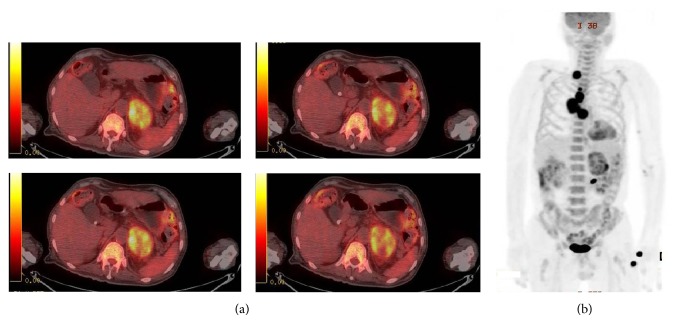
Transaxial image of ^18^F FDG PET/CT showed heterogeneously FDG-avid left adrenal gland tumor (a) and MIP of ^18^F FDG PET/CT (b) also revealed multiple FDG-avid lymphadenopathies in mediastinum and right supraclavicular area.
